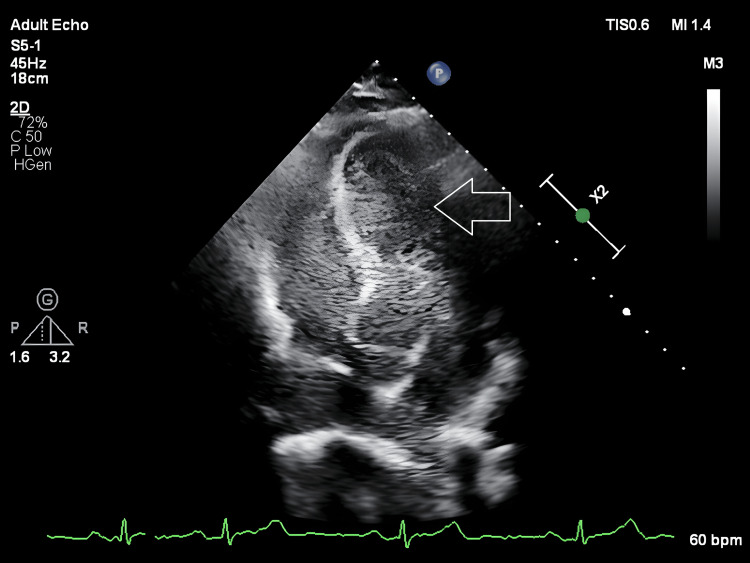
A still image from bubble echocardiography showing opacification of all four chambers (arrow).
